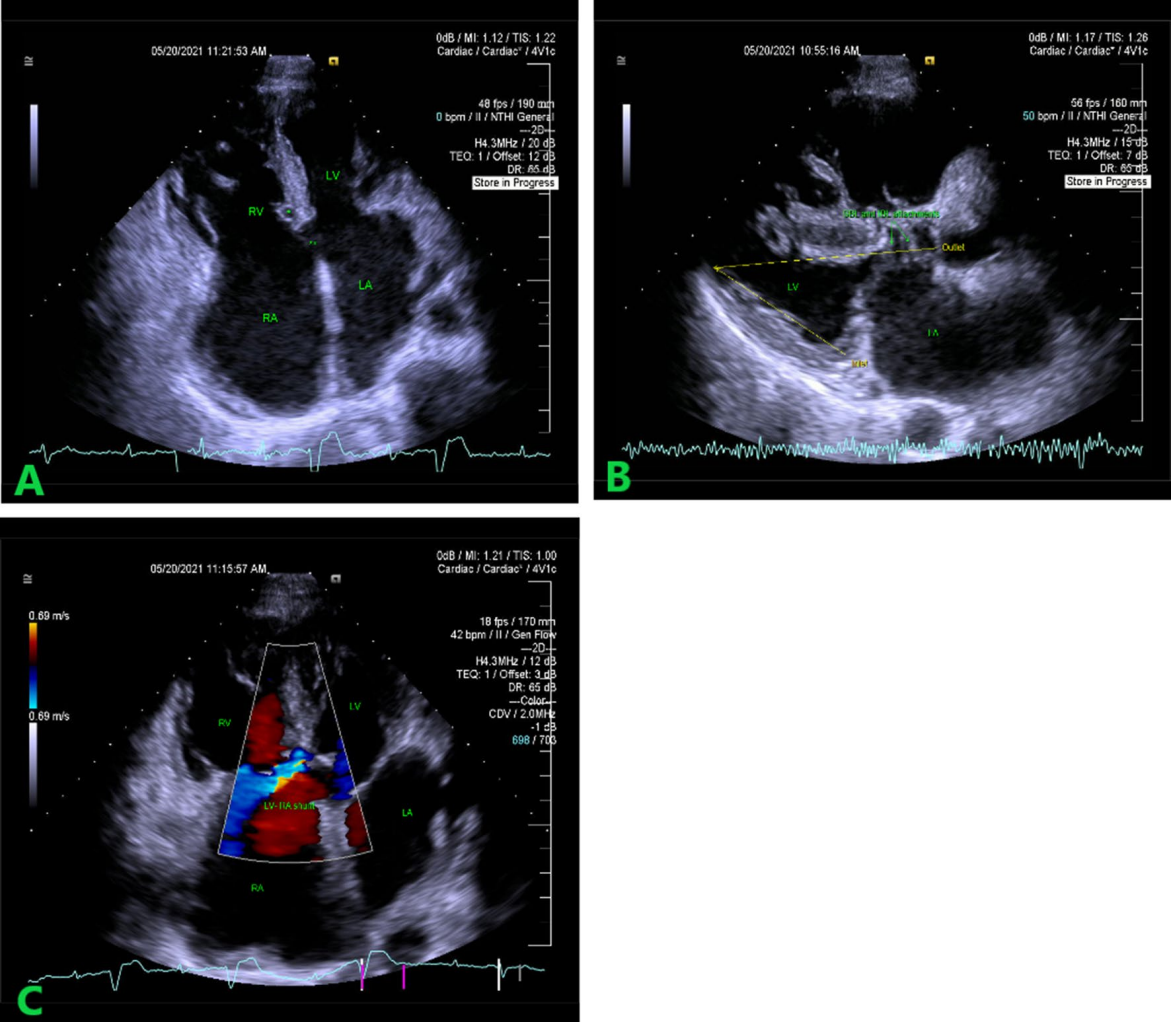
A Atrioventricular septal defect. 2D TTE—apical four chamber view. AV septum deficiency (double-asterisk). Right heart chambers enlargement. Both AV valves are placed at the same level. The tricuspid pouch (asterisk). B Left ventricular outflow tract prolongation. Parasternal long axis view. Larger outlet dimension compared to the inlet portion distance. Hemodynamically insignificant obstruction of LVOT, caused by the attachment of the bridging leaflets. C Left ventricle to right atrium shunt due to AV septum insufficiency. Color flow-mapping imaging. *TTE* transthoracic echocardiography, *AV* atrioventricular, *LVOT* left ventricular outflow tract
